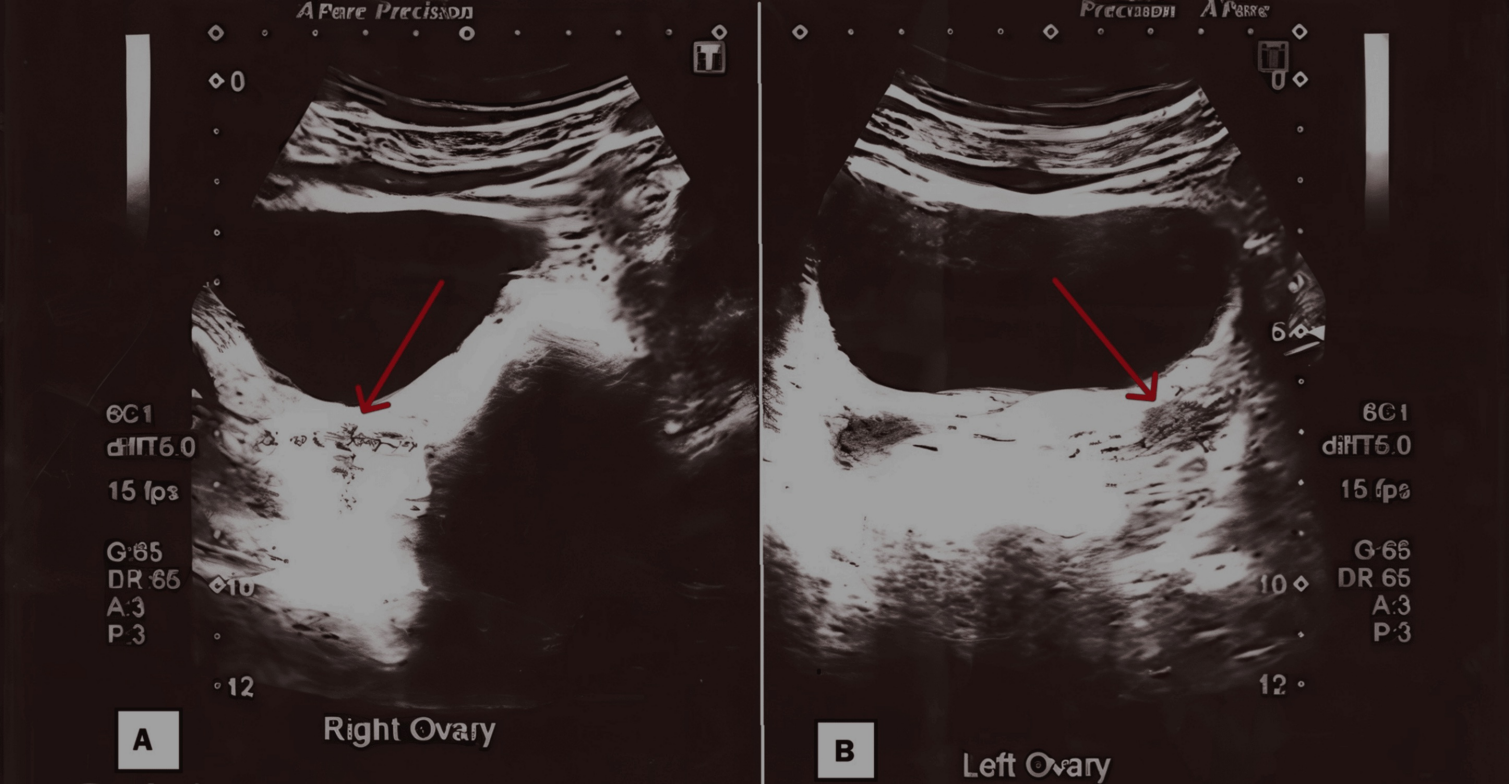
Ultrasound of the pelvis showing streak ovaries (A, B). Red arrows point towards the streak ovaries. A: Right Ovary, B: Left Ovary.
